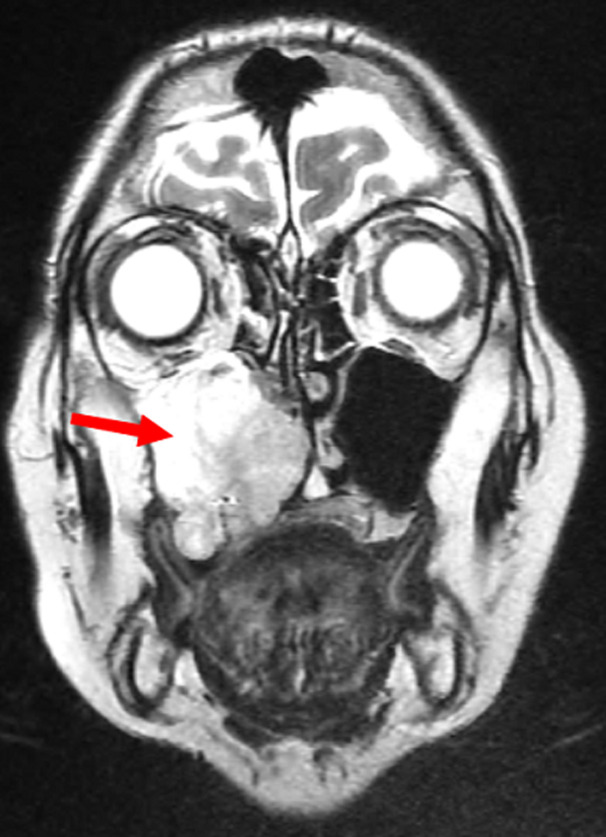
coronal section of the magnetic resonance imaging showing a tissue lesion in the right maxillary sinus (red arrow) extending into the endobuccal region and the ipsilateral nasal cavity
